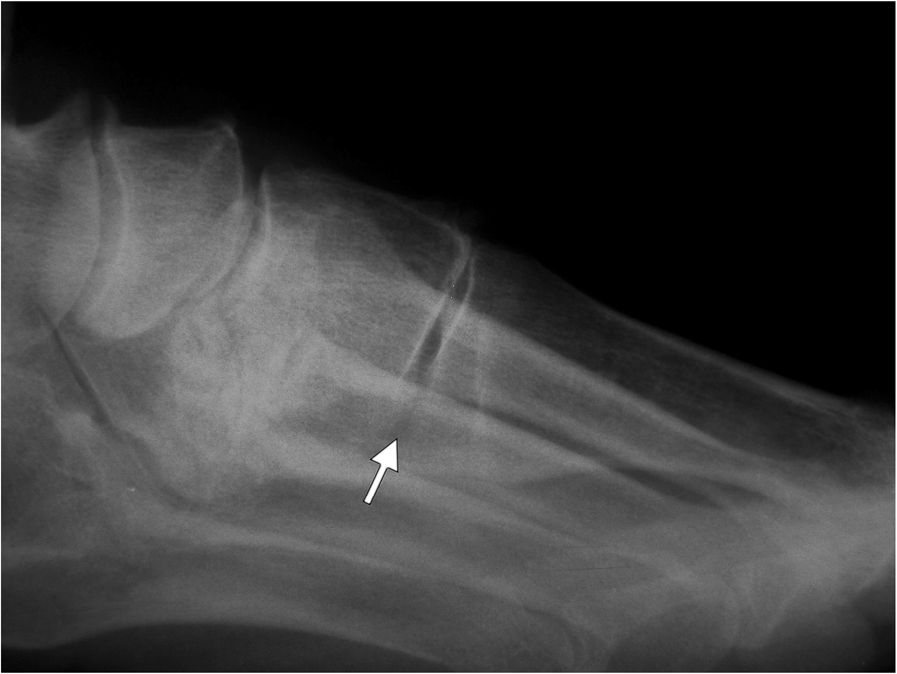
Plantar gapping of medial cuneometatarsal joint. Lateral X-ray weight bearing picture shows the plantar gapping (wedging) of the medial cuneometatarsal joint (white arrow).
